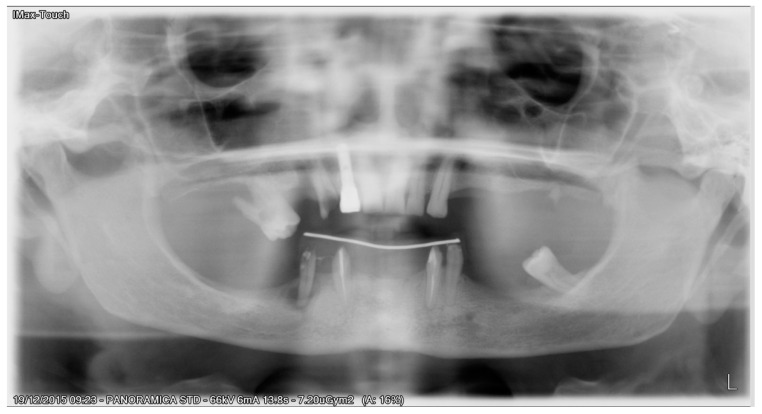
Preoperative panoramic X-ray.
